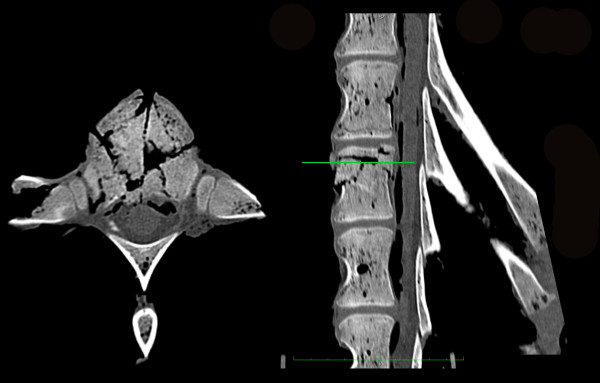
CT scans showing example of the produced incomplete burst fracture in calf spine samples.
